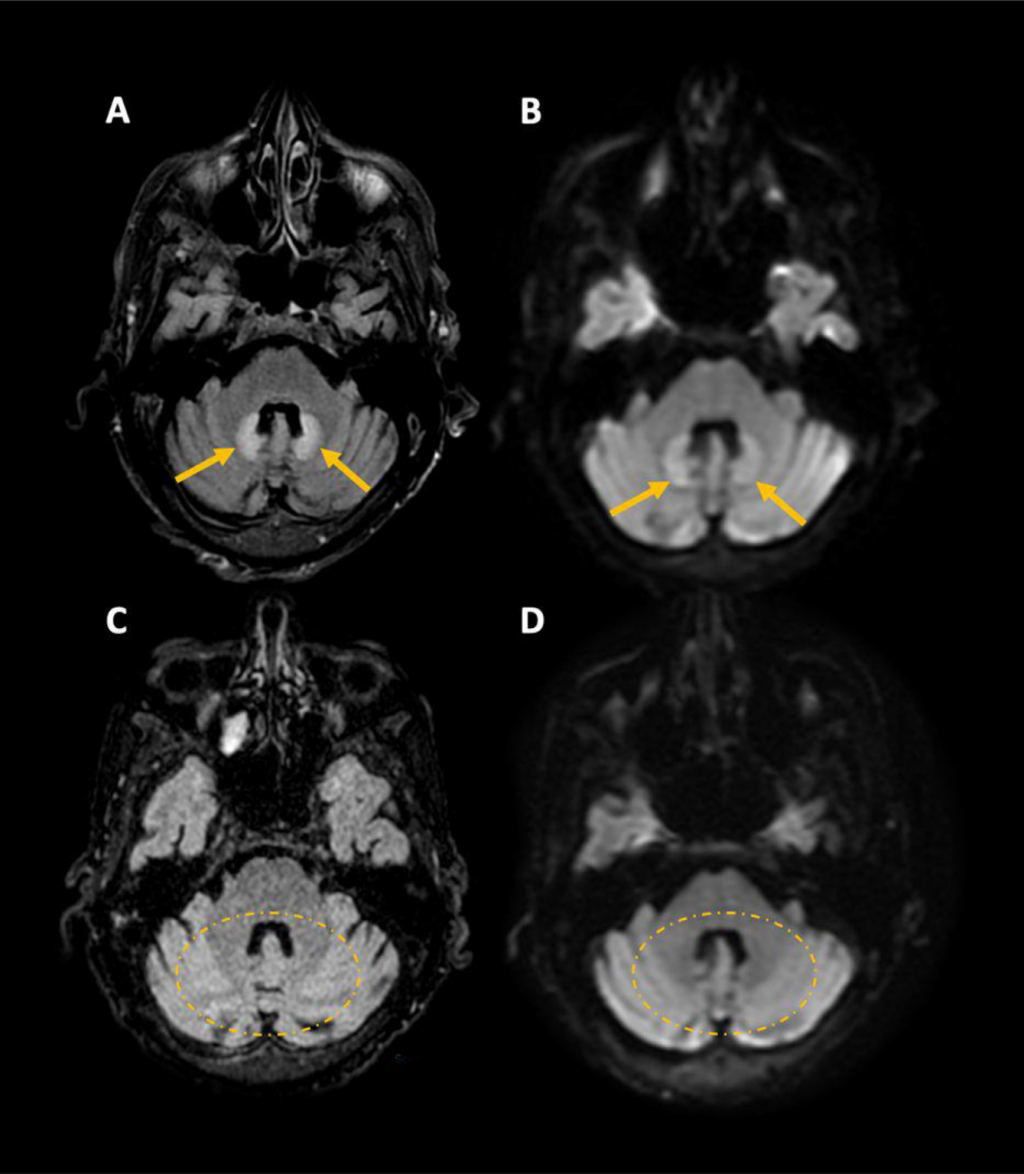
Axial FLAIR (A and C) and DWI (B and D) sequences of a brain MRI. At the initial presentation, there was symmetric FLAIR/diffusion hyper signal in the dentate nuclei bilaterally (arrows). Follow-up MRI 3 months after discontinuation of the medication demonstrated complete resolution of the lesions, without sequelae (dashed circles).
